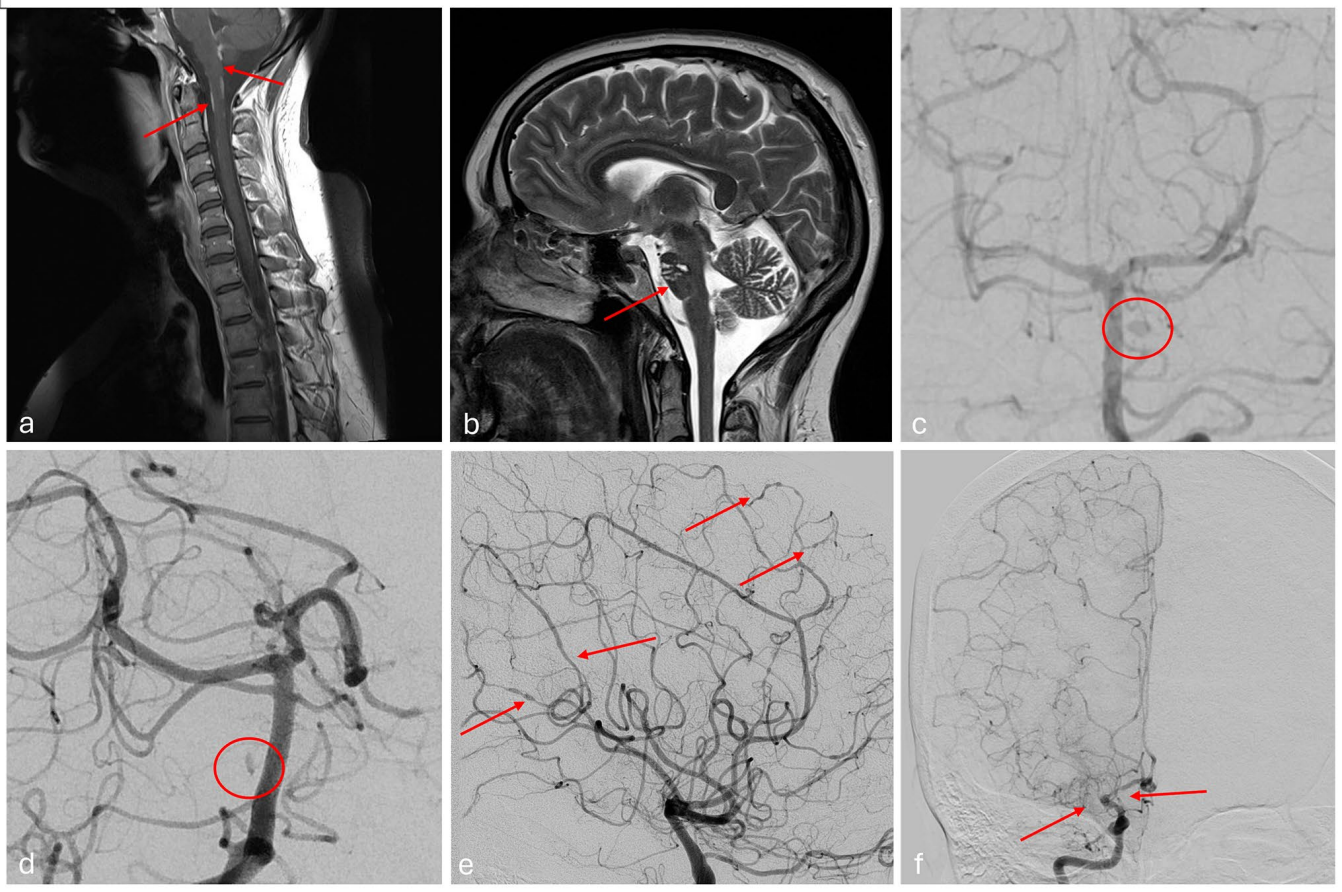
Neuroradiological characteristics of 4 patients with cerebral vasculitis related to neurosarcoidosis a MRI with leptomeningeal gadolinium enhancement in patient 1 from Table 5. b MRI with gliosis of brainstem in patient 1 from Table 5. c Angiogram with one microaneurysm of a pontine perforator artery in patient 1 from Table 5. d Angiogram with one microaneurysm of a pontine perforator artery in patient 4 from Table 5. e Angiogram with bilateral multiple caliber irregularities in patient 2 from Table 5. f Angiogram with moyamoya-like vasculitis in patient 3 from Table 5
